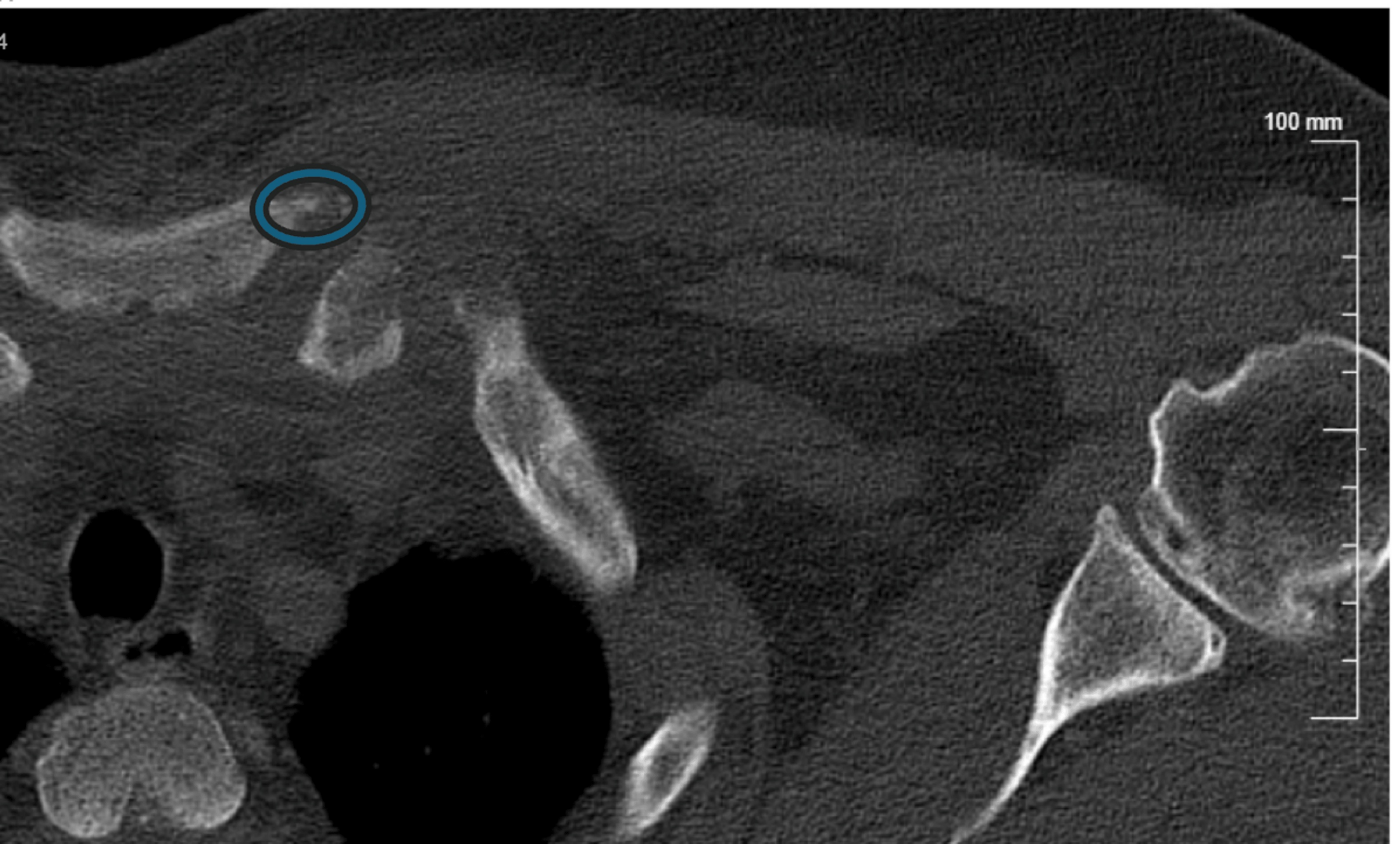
CT scan (bone window) The axial CT scan with bone window at the level of the sternoclavicular joint demonstrates cortical irregularity and erosive changes involving the medial (sternal) end of the left clavicle. The blue circled region highlights cortical disruption and periarticular erosion on the clavicular side, with adjacent soft tissue swelling suggestive of inflammatory extension beyond the joint.
